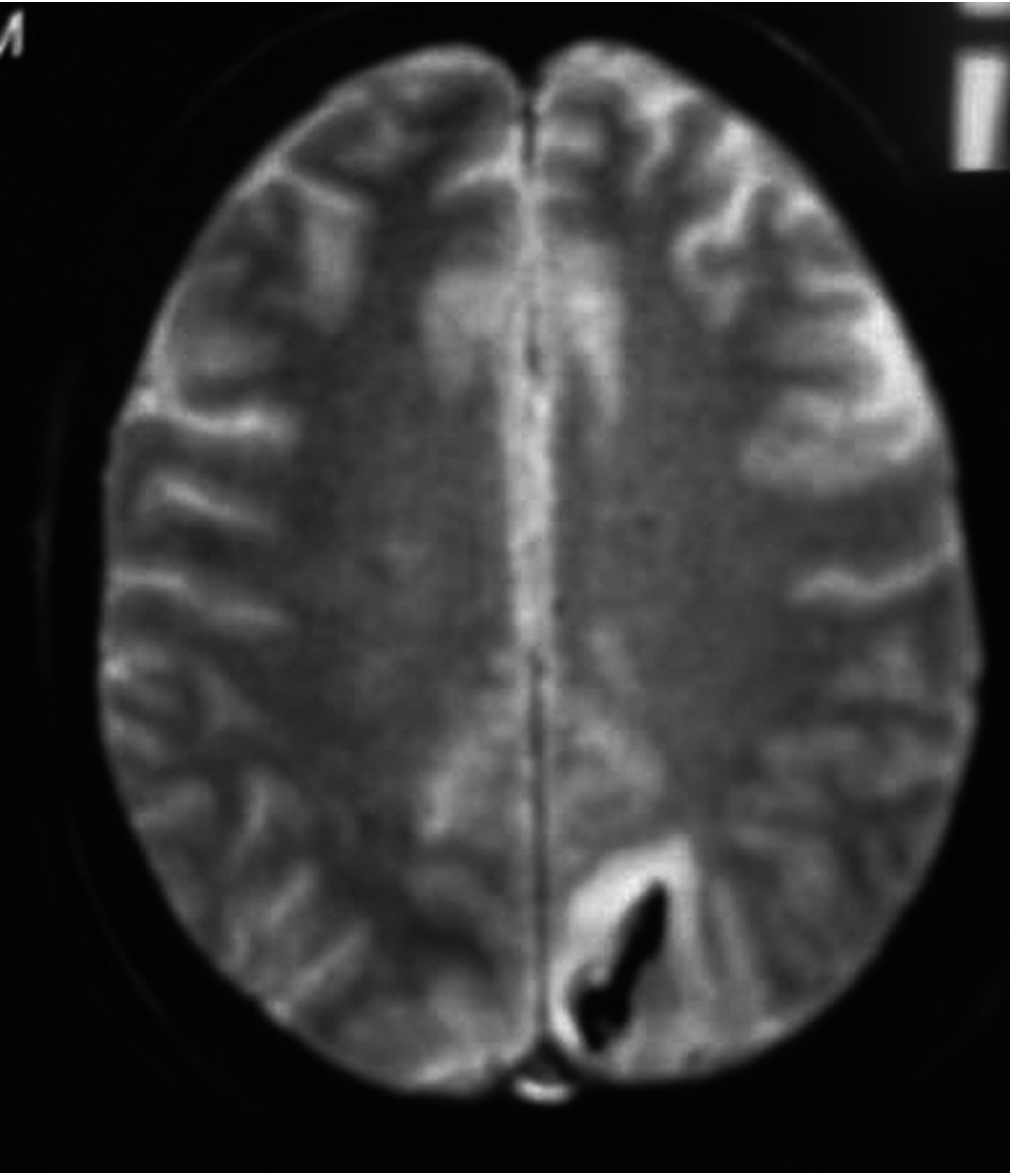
Brain MRI GRE: left subcortical parieto-occipital hemorragic foci.
